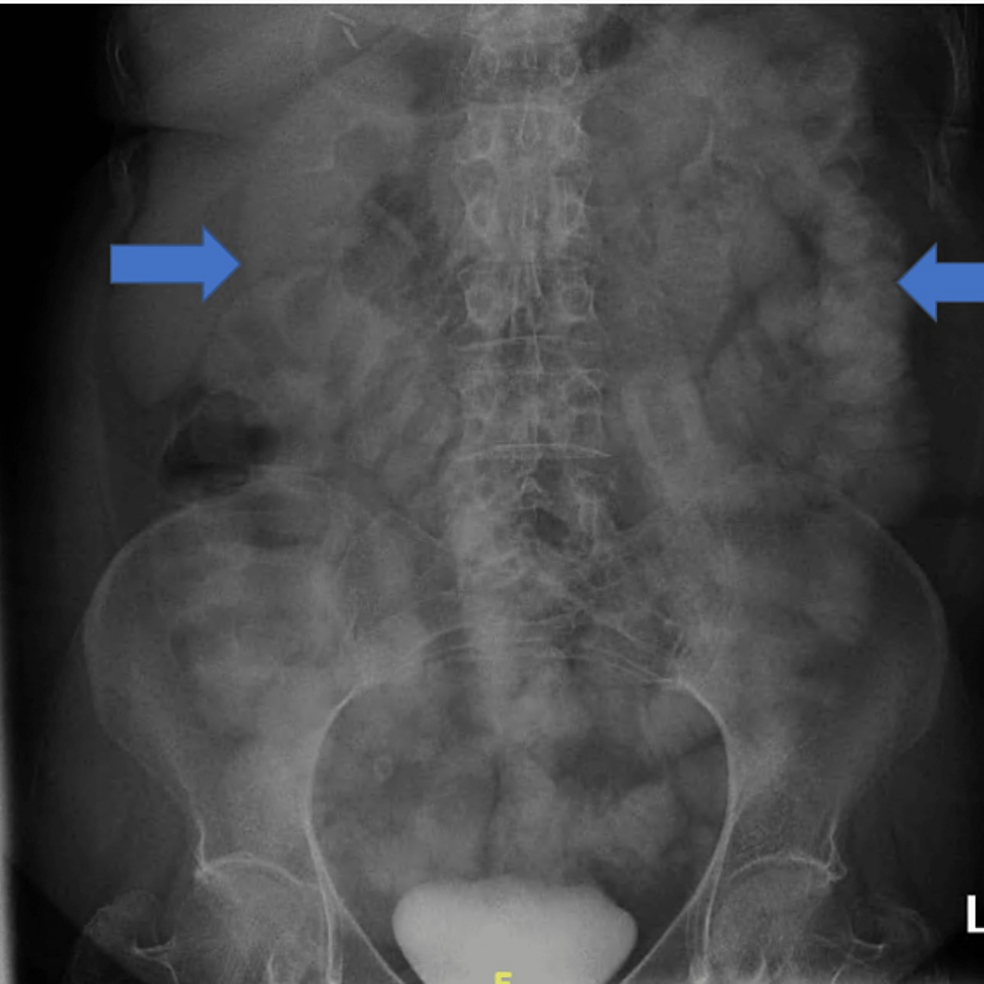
X-ray of the abdomen with gastrograffin There is contrast throughout the small and large bowel with some dilated small bowel loops, the largest measuring 3.5 cm. No free air or abnormal calcifications are seen.
